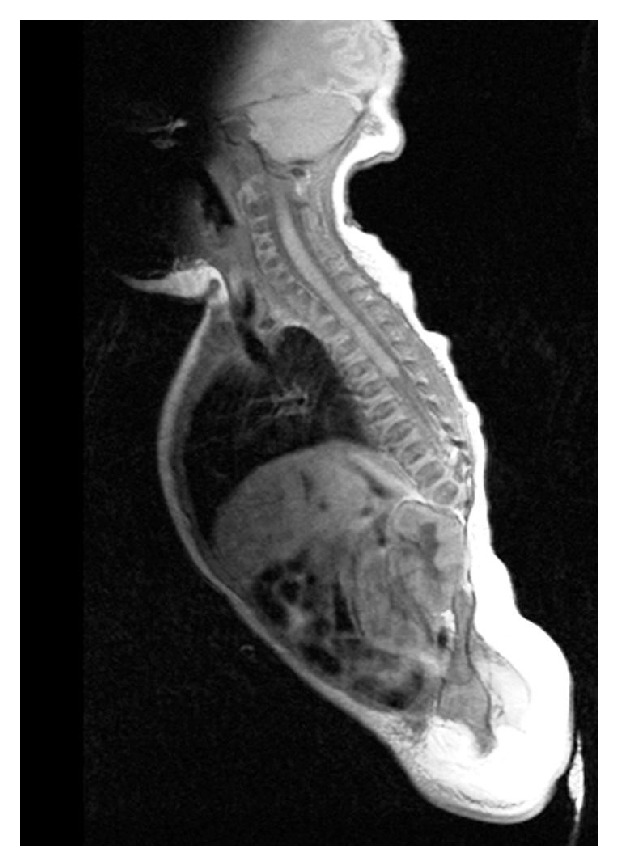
Sagittal T2W MRI of the spine shows severe caudal regression with complete absence of the lumbar spine and sacrum. The conus medullaris has a characteristic, abnormal, wedge-shaped (blunted) appearance.
